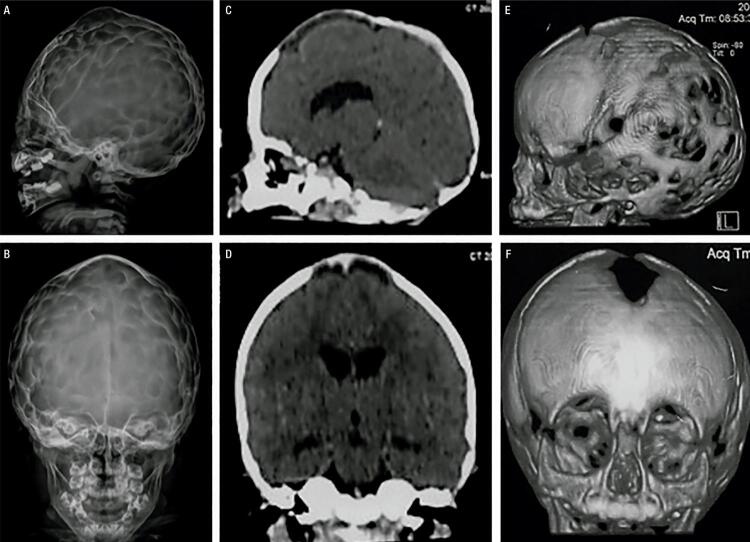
Skull images at the age of 1 year and 8 months. (A-B) Radiographs showing the “beaten copper sign”. (C-F) Cranial computed tomography (CT) images showing misshapen skull, digitiform impressions, and prominence of the brain parenchyma at the anterior fontanelle.
